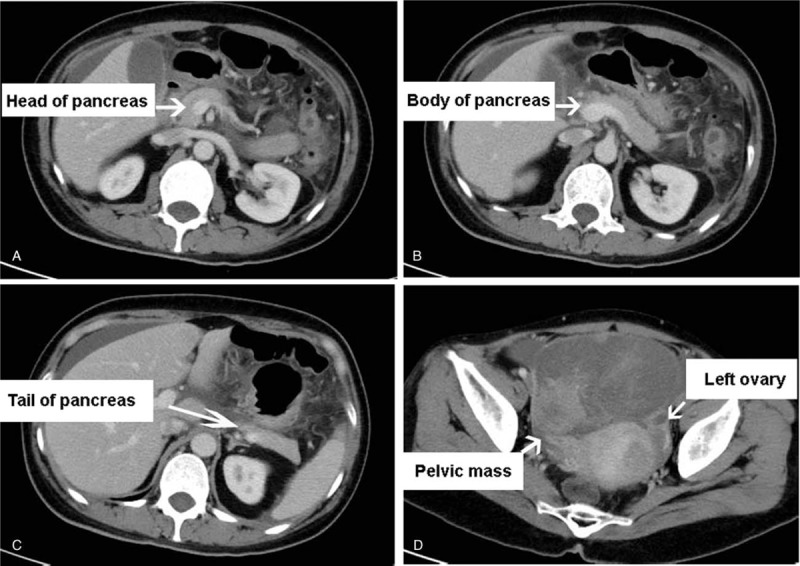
Computed tomography (CT) image of the abdomen and pelvis: a 12 cm-sized solid mass with uneven density in the pelvis (D). Normal image of the pancreas (A–C). CT = computed tomography.
